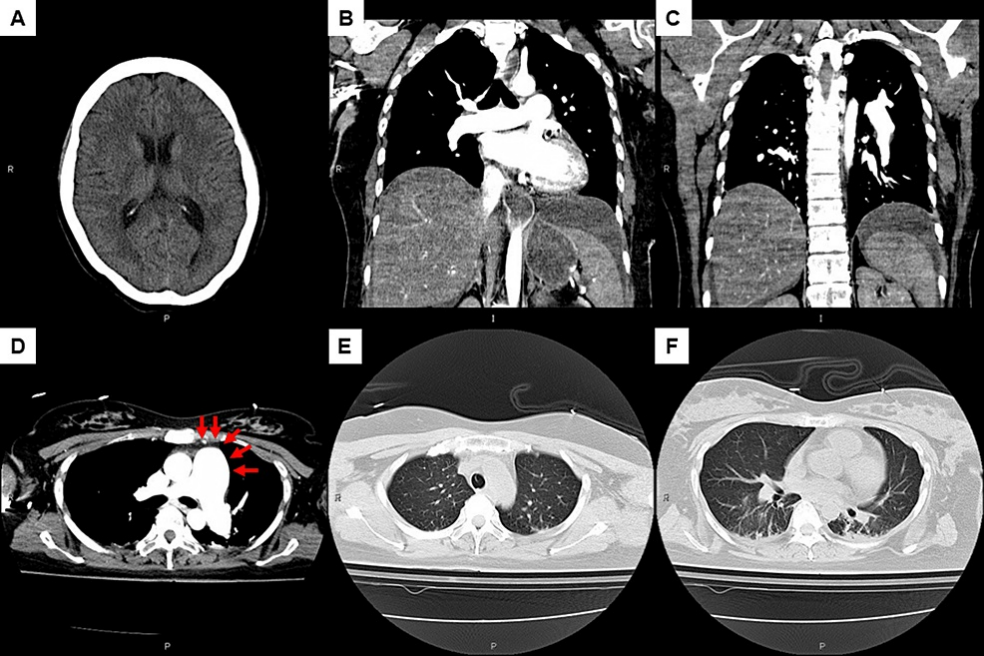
Head and chest computed tomography with contrast media one hour after the onset of dyspnea with hypotension. The patient had no evidence of intracranial hemorrhage (A), pulmonary thromboembolism (B–D), pneumothorax, pneumonia, or significant amount of pleural effusion (E, F). The pulmonary artery trunk was significantly dilated compared to the adjacent aorta (D, arrows), which suggested pulmonary hypertension.
